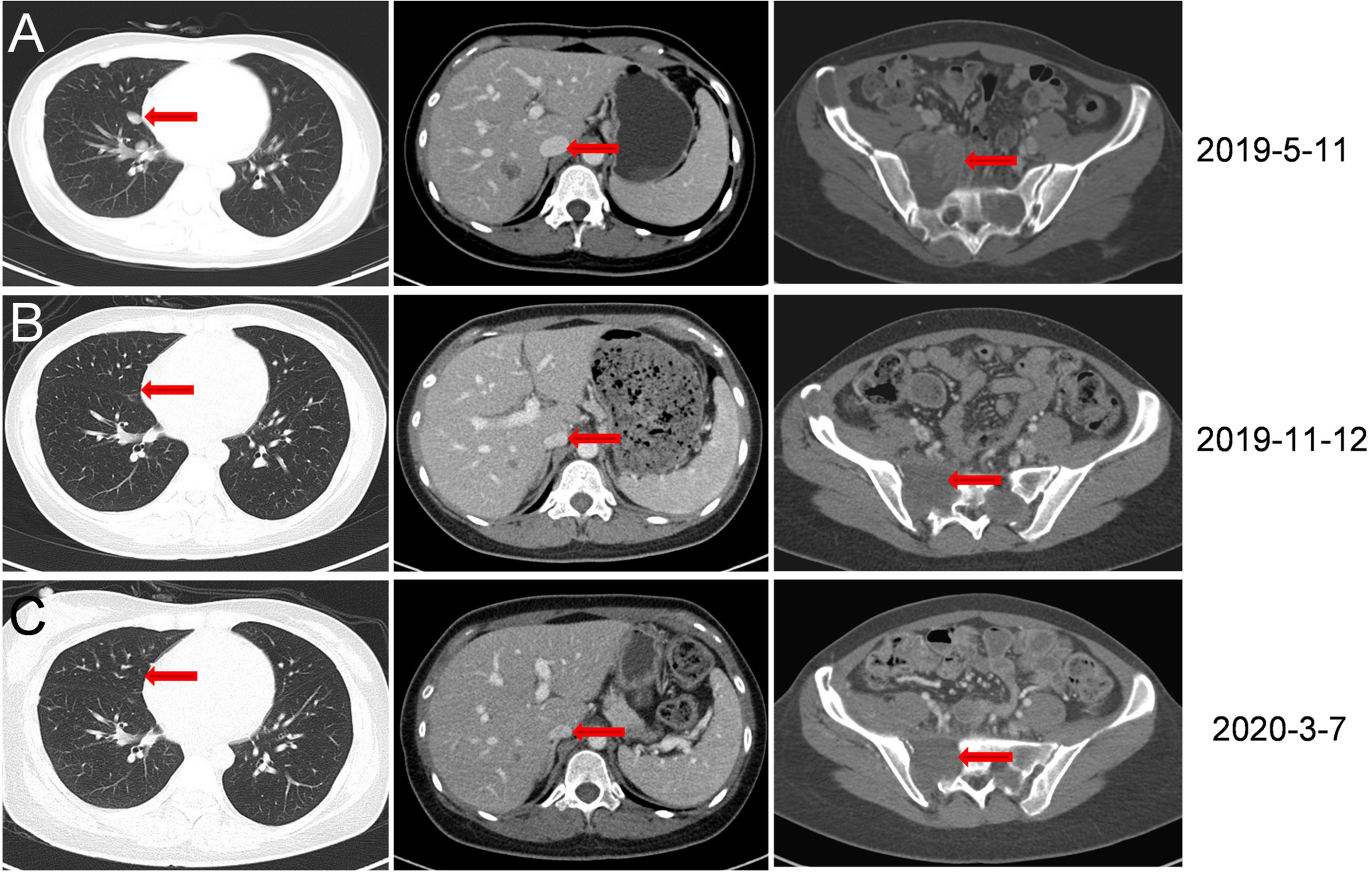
CT scan images during treatment. (A) In May 2019, eight months following anlotinib treatment, new multiple lung metastases were observed. (B) In November 2019, six months following anlotinib combined with toripalimab treatment, lesions in the lung disappeared. Liver and sacral vertebrae metastases shrank. (Size was reduced from 5.5 cm × 5.4 cm to 3.5 cm × 3.8 cm). (C) In March 2020, CT re-examination indicated stable disease.
